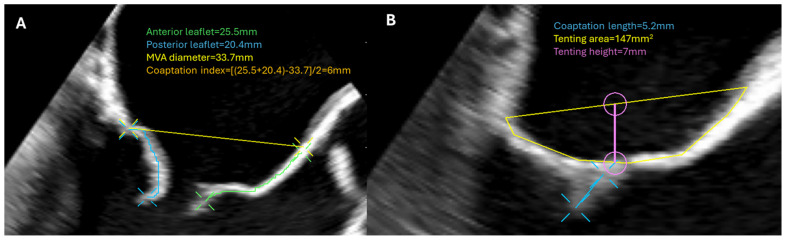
Essential echocardiographic measurements in patients with MR. (A) In the left panel is a patient with severe primary MR. Measurement of the leaflet length and annulus diameter as well as the calculation of the coaptation index can predict a sufficient coaptation length after an MV repair operation. These measurements are more important in operations without the availability of annular ring placement, as is the case in transapical chord implantation interventions. (B) In the right panel, there is a patient with moderate to severe secondary MR. Increased tenting area and tenting height are associated with increased recurrence rate and worse prognosis after isolated annuloplasty. MR: mitral regurgitation; MV: mitral valve; MVA: mitral valve area.
